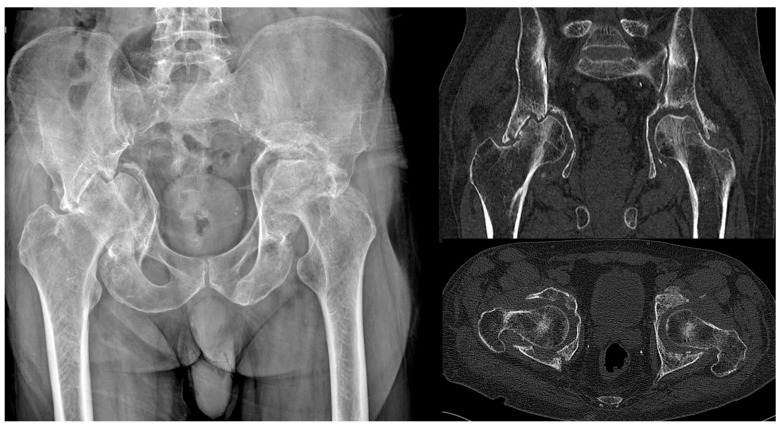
Anteroposterior pelvic radiograph and CT scans showing bilateral acetabular impaction fractures in a patient who sustained a high-energy fall. The patient refused acute treatment. The imaging demonstrates the condition three months post-injury.
